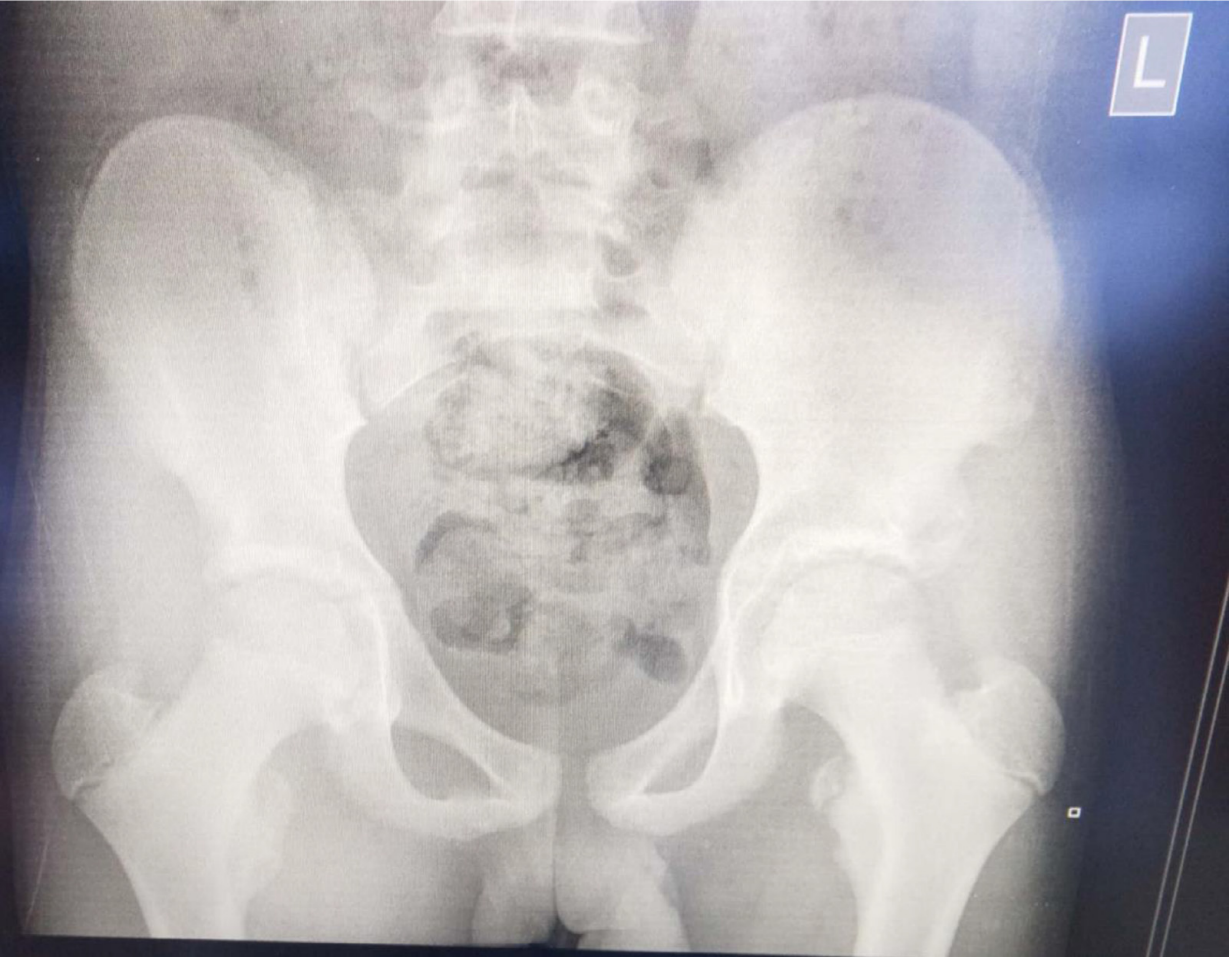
Radiograph of the pelvis 9 weeks following conservative and nonsurgical management of left lesser trochanter avulsion injury.
